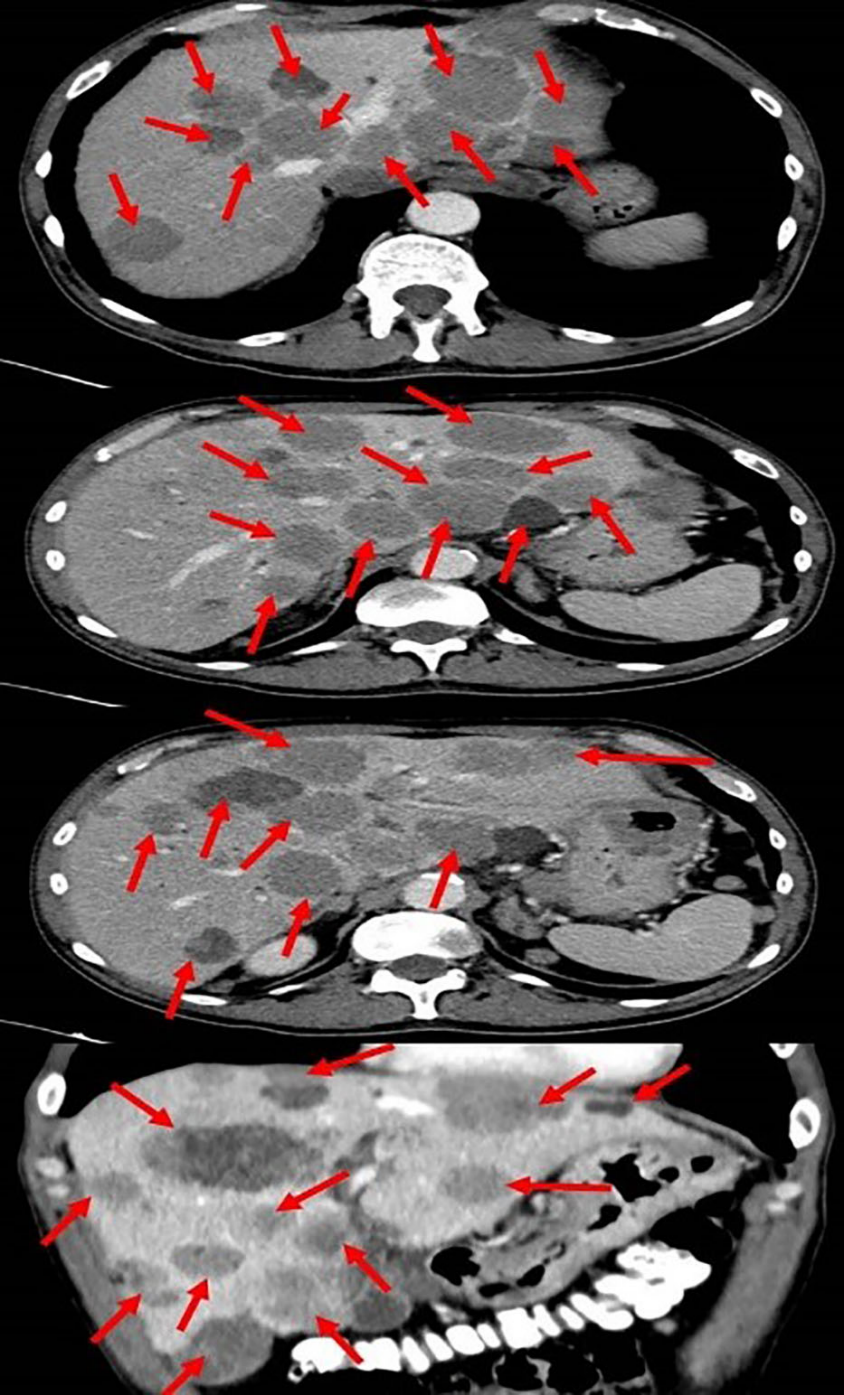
Total body computed tomography scan showing the metastatic melanoma in the liver (red arrows).
